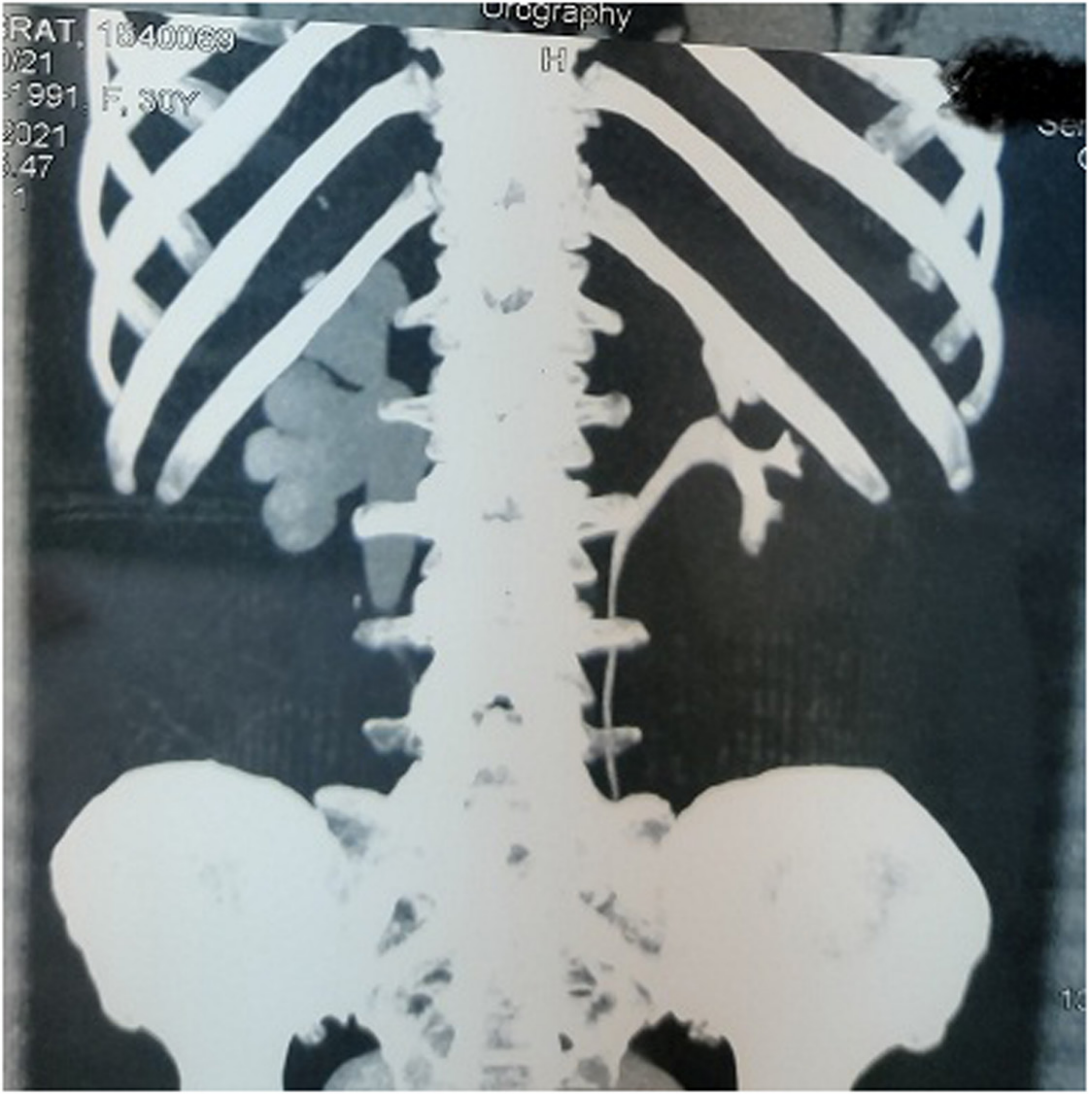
Computed tomography urography: reconstructed image showing right-sided hydroureteronephrosis due to upper ureteric stricture.
